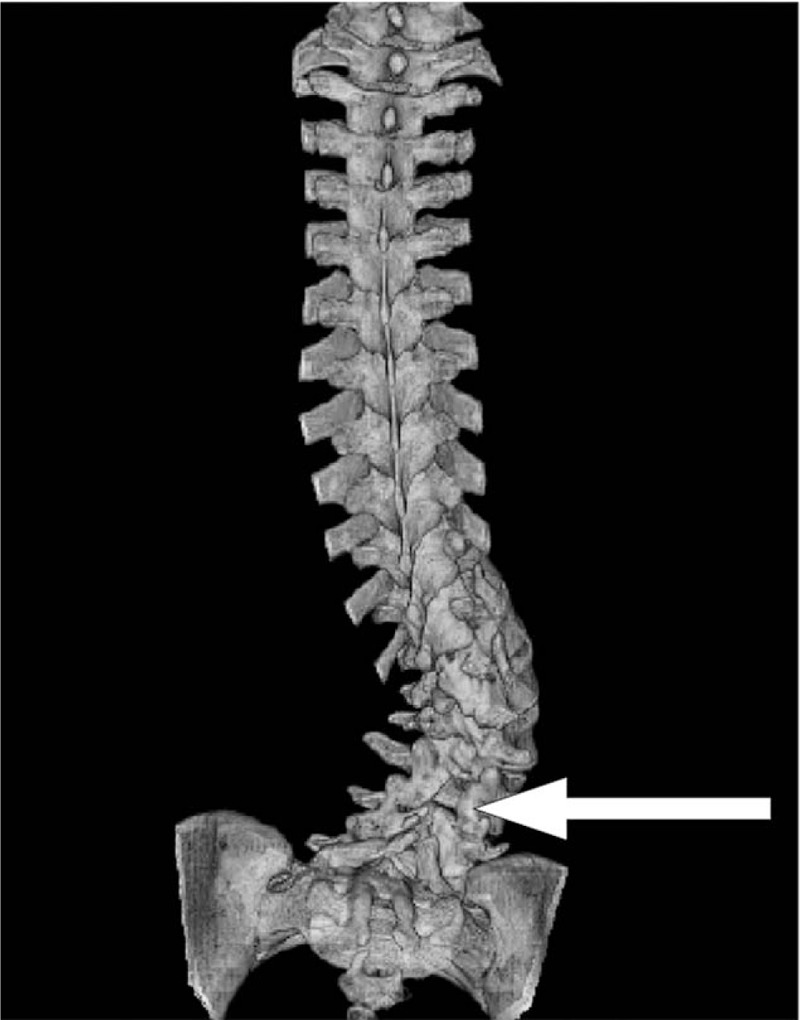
Preoperative computed tomography image showing spina bifida, from L2 to the sacrum.
